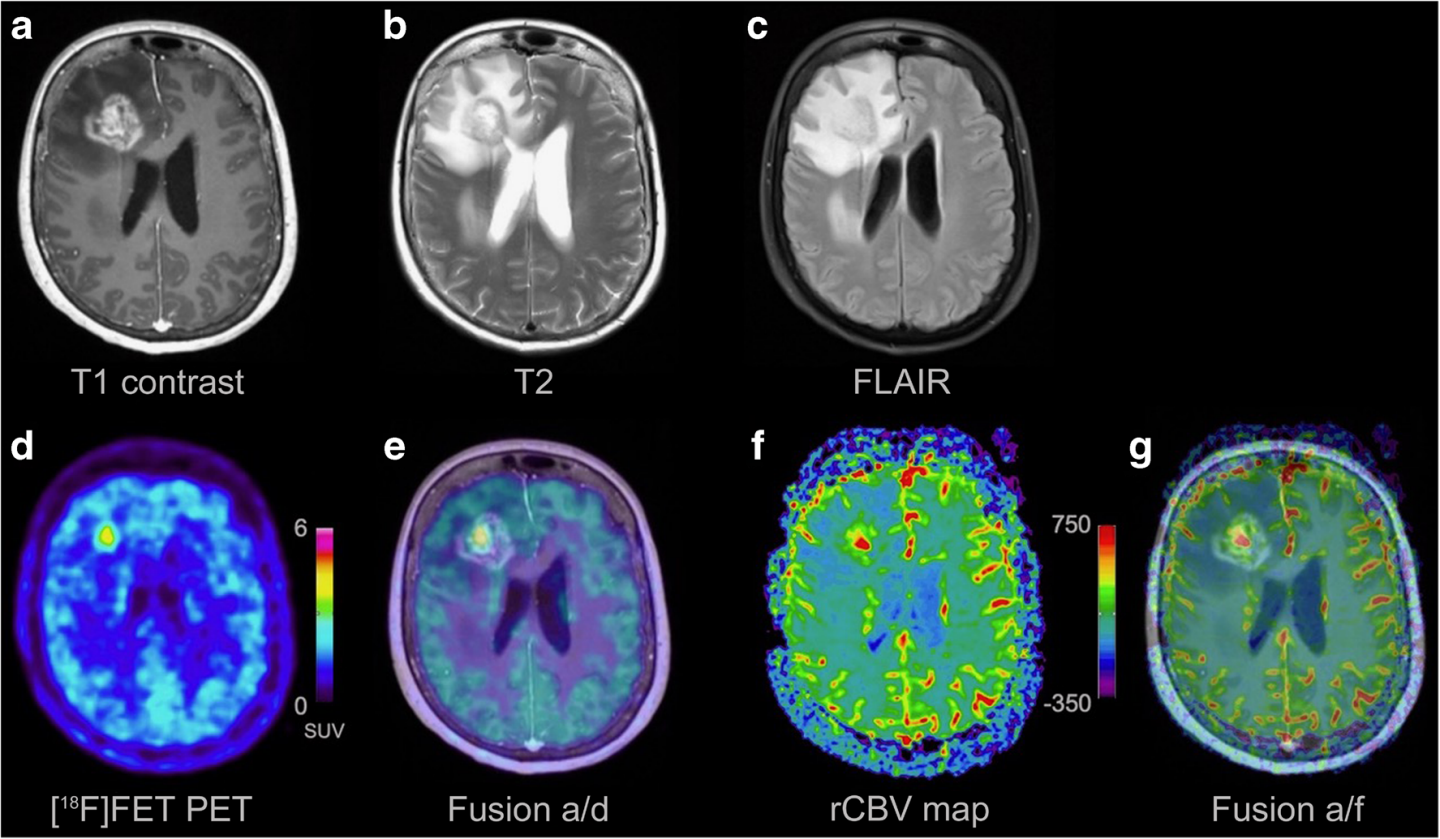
Example of corresponding PWI and [^18^F]FET PET. A T1-weighted contrast-enhanced (a), a T2-weighted (b), and a fluid-attenuated inversion recovery (FLAIR, c) MR image of a patient with tumor progression (TP). The corresponding [^18^F]FET PET (map only shown in d; map as transparent overlay on the T1-weighted contrast-enhanced image shown in e) and PWI-derived cerebral blood volume map (CBV; map only shown in f; map as transparent overlay on the T1-weighted contrast-enhanced image shown in g) demonstrate a hotspot in the right frontal lobe
